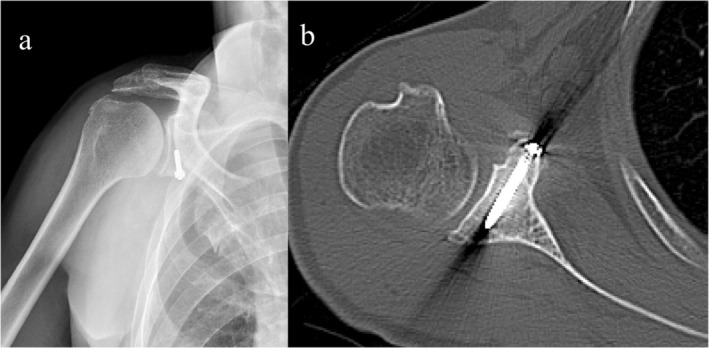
Preoperative imaging findings. (a) Plain radiograph showing irregularity of the greater tuberosity and mild acromial acetabularization without osteonecrotic change. (b) CT demonstrating bony union of prior coracoid transfer with embedded screw fixation.
